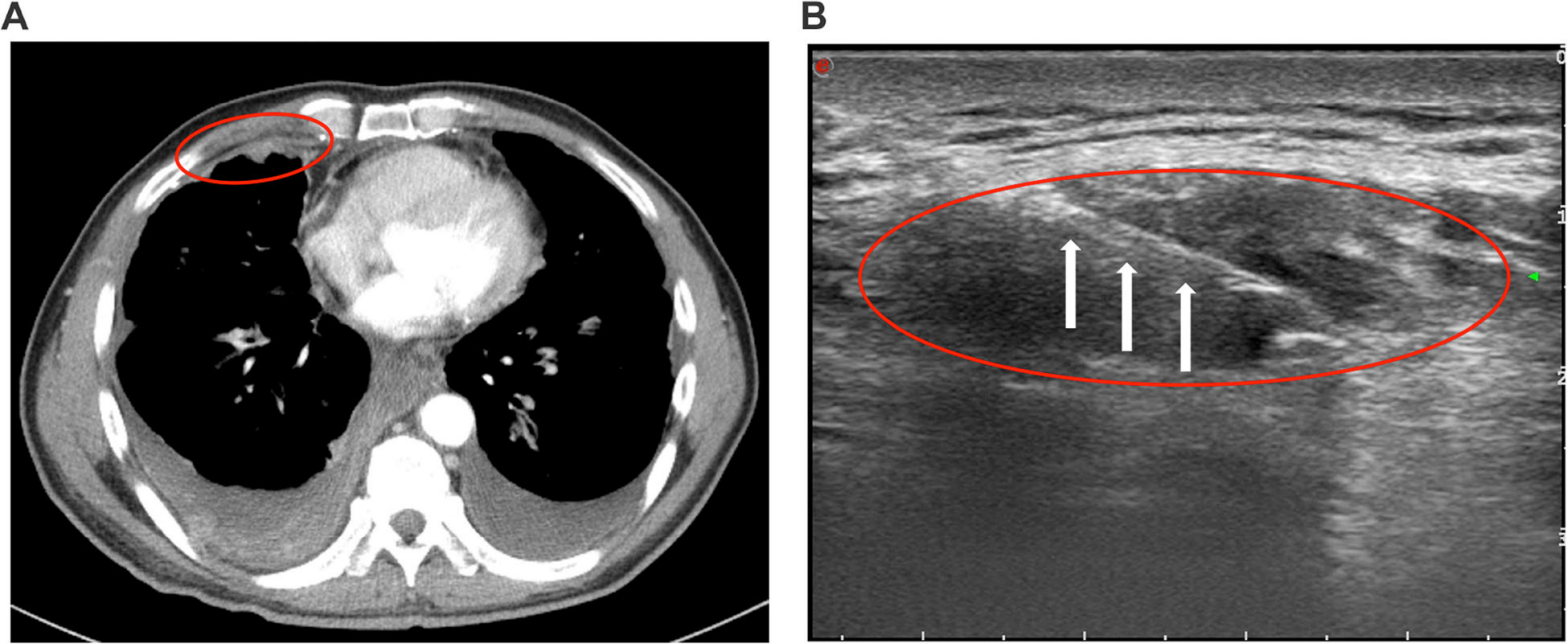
Typical CT scan and ultrasound imaging of patients with pleural thickening. (A) CT scan, red oval refers to the pleural thickening area; (B) Ultrasound imaging, red oval refers to the pleural mass, white arrows point to the biopsy needle
